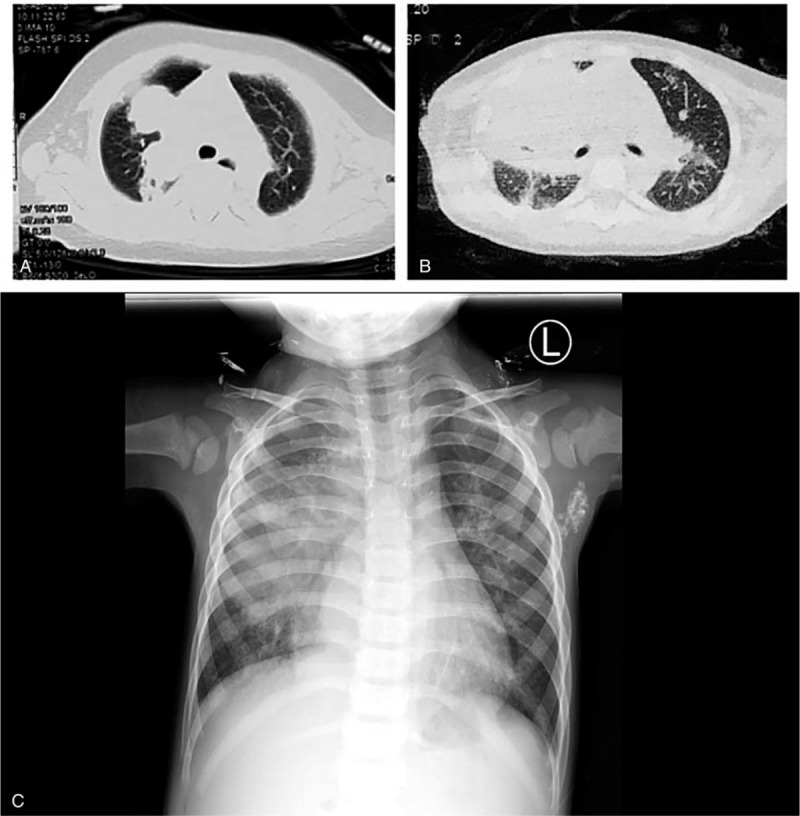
Patient #2, a boy of 4 months and 28 days, admitted to the hospital for cough for over 2 months and aggravated for 4 days. (A-B) Thoracic computed tomography showing multiple nodular high-density shadows or mass-like shadows. (C) Thoracic X-ray image 2 years later, showing calcification of the left axillary lymph nodes.
